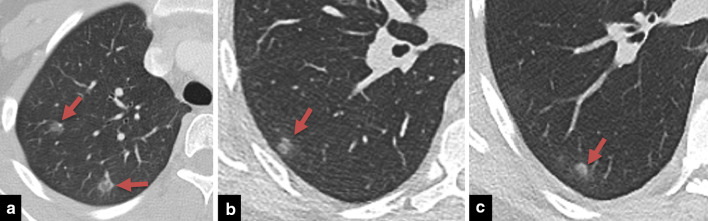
Eosinophilic pneumonia and COVID-19 pneumonia. a Nodular GGOs are seen in the right upper lobe in a patient diagnosed with eosinophilic pneumonia (arrows). b, c Axial CT images of a patient with COVID-19 show nodular GGOs in the right lower lobe (arrows)
